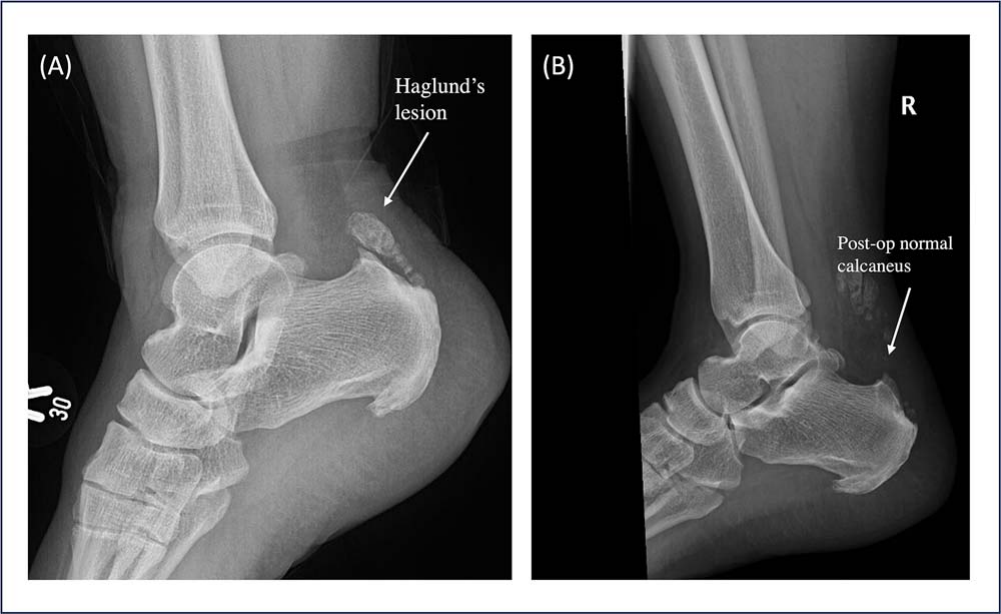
Pre- and postoperative lateral ankle X-ray of Patient 5. (A) Pre-operative shows a prominent Haglund’s lesion before surgery. (B) The postoperative image demonstrates a normalized calcaneal contour following debridement and Achilles tendon repair, suggesting adequate resection and restoration of the tendon insertion profile.
